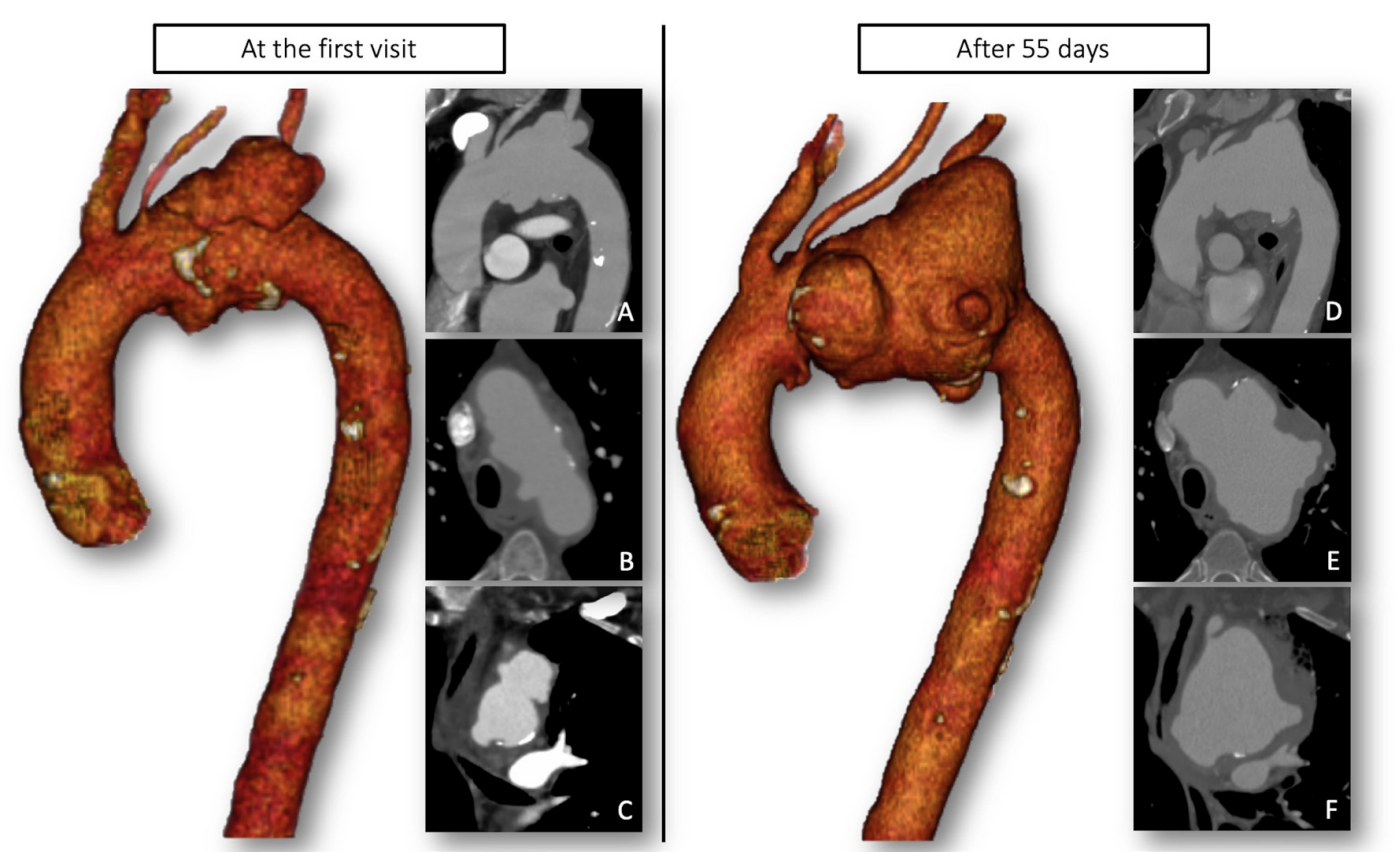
Preoperative computed tomography angiography (CTA) showing rapid growth of the syphilitic aortic arch aneurysm 55 days after the first visit to the outpatient’s clinic (A, B, C) and the urgent setting. (D, E, F) Sagittal (A/D), axial (B/E), and coronal (C/F) views of the two preoperative CTAs, almost two months apart.
